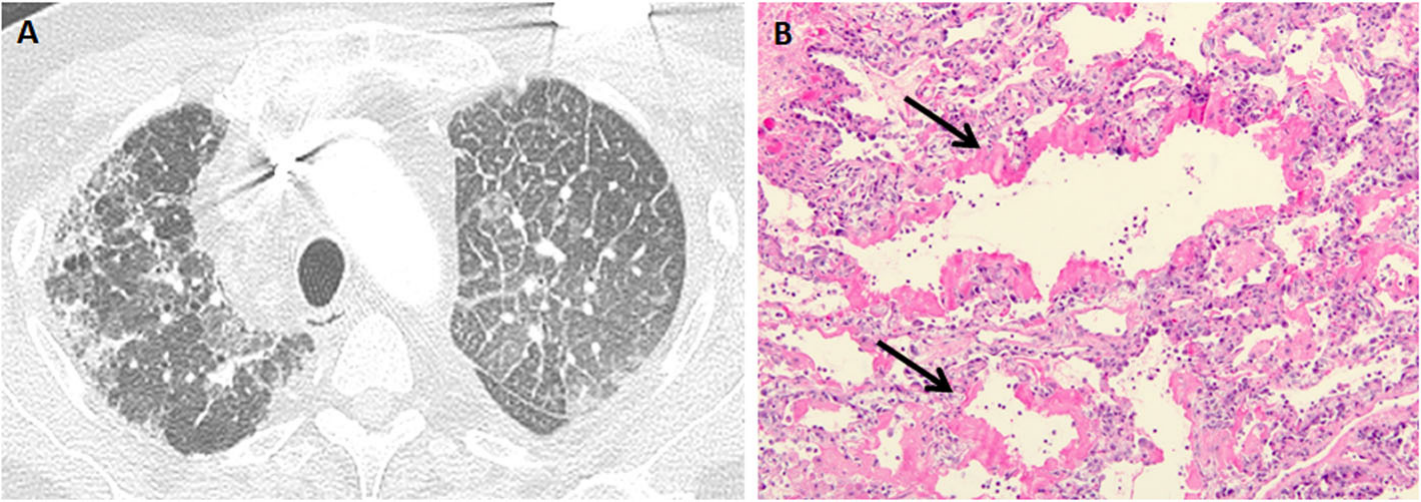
Primary graft dysfunction: imaging and transbronchial biopsy findings. The patient was 2 days status-post left lung transplant and developed increasing hypoxemia. Axial CT images (a) shows smooth interlobular septal thickening with ground-glass opacities in the transplanted left lung. These findings are typical of primary graft dysfunction but are indistinguishable from acute rejection. Transbronchial biopsy (b) from a different lung transplant patient with primary graft dysfunction showing diffuse alveolar damage. Note hyaline membranes (arrows)
